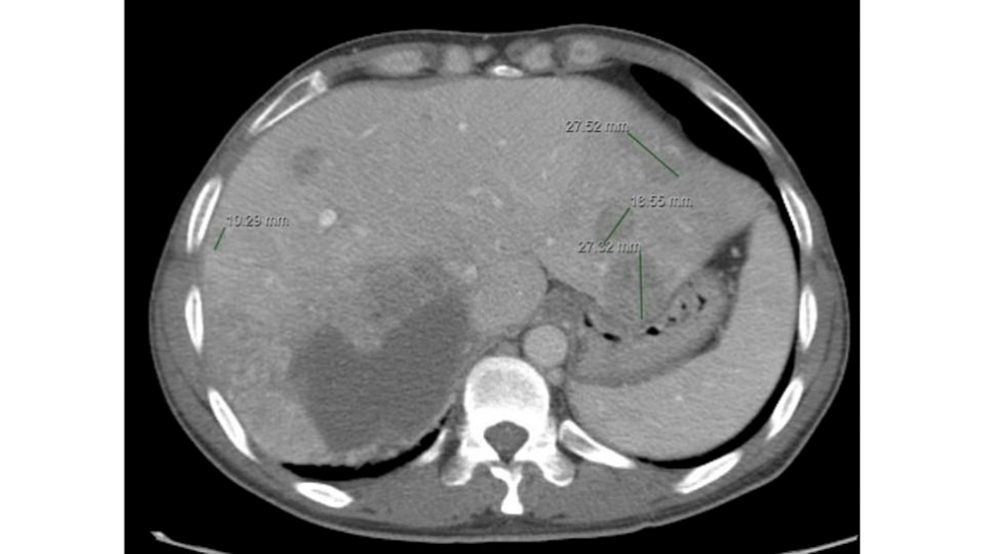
Axial view of the CT scan demonstrates multiple hepatic lesions in the left and right hepatic lobes.
